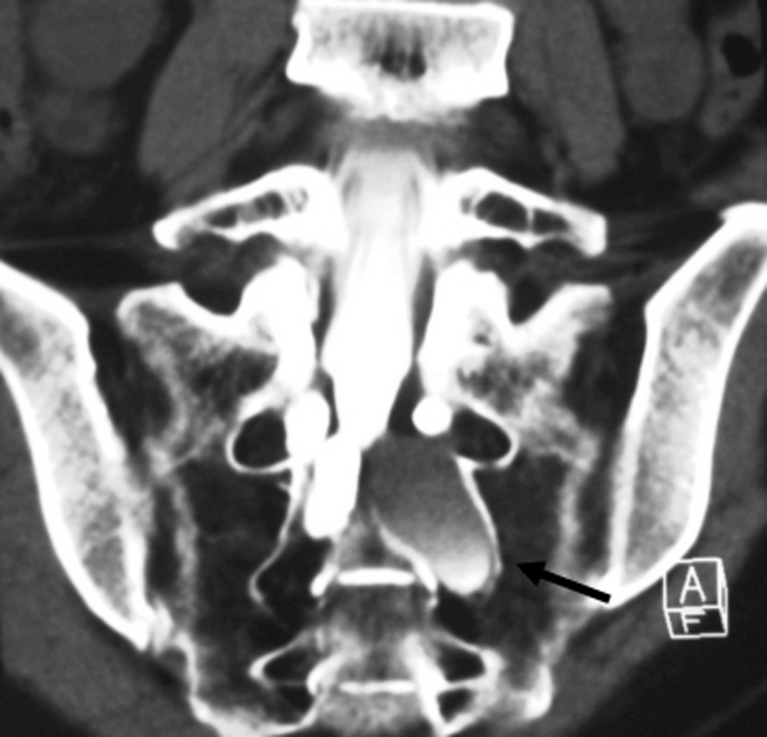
Coronal CT myelography in case 6, allowing easy identification of primary cyst (*arrow*)
